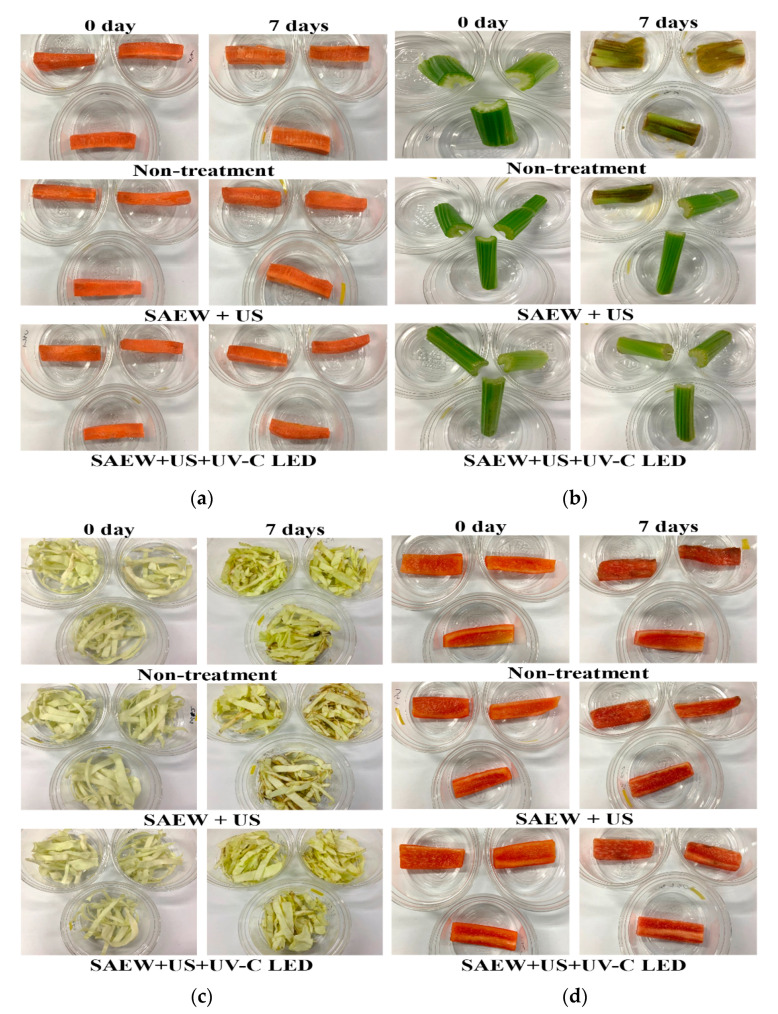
Effect of combined treatments on visible qualities in various fresh-cut vegetables after storage for 7 days at 15 °C. (a) Carrot, (b) celery, (c) cabbage, (d) paprika; non-treatment, no extra wash with a sanitizer; SAEW, slightly acidic electrolyzed water; US, ultrasounds; UV-C LED, ultraviolet-C light-emitting diodes (275 nm).
